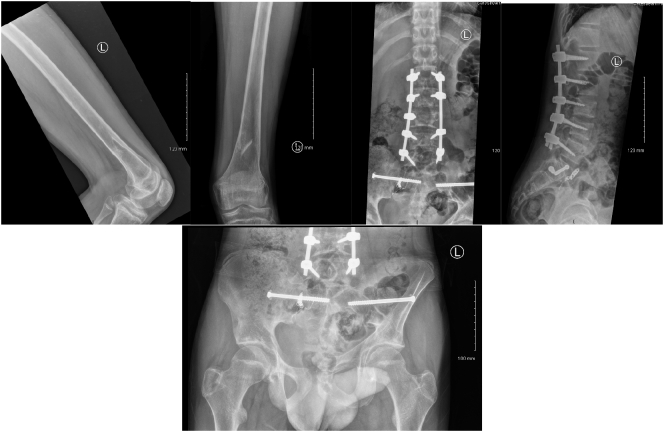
post-operative radiograph (after one year) of the lumbar spine, pelvis and left femur shows satisfactory healing and alignment.
